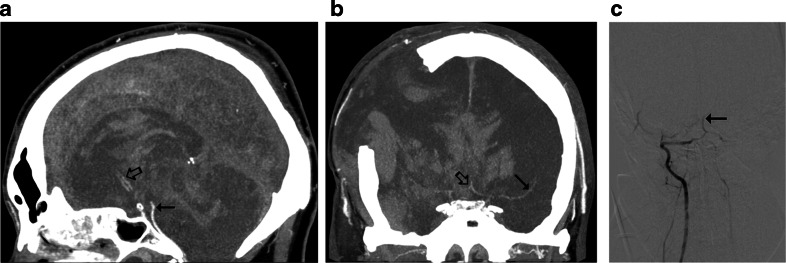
CTA findings in a 50-year-old man (patient no. 45) with traumatic brain injury (epidural hematoma in the right parietal region, massive intracerebral, and subarachnoid and intraventricular hemorrhage) and right sided craniectomy presented with signs of BD on clinical examination: a Ten millimeter maximum intensity projection (MIP) in sagittal plane. CTA shows opacification of the BA (*thin arrow*) and a trace of contrast in A2 segments of the ACAs (*thick arrow*). b Ten millimeter MIP in coronal plane. CTA shows opacification of the M1 segment of the left MCA (*thin arrow*) and the A1 segments of the ACAs (*thick arrow*); these findings exclude the diagnosis of BD according to the 10-point scale but confirm BD according to the 7- and 4-point scales. c Catheter angiography of the right VA performed 0.5 h later revealed delayed, residual filling of the BA (*arrow*) that occurred 21 s after injection. This result was interpreted as stasis filling consistent with the diagnosis of BD
